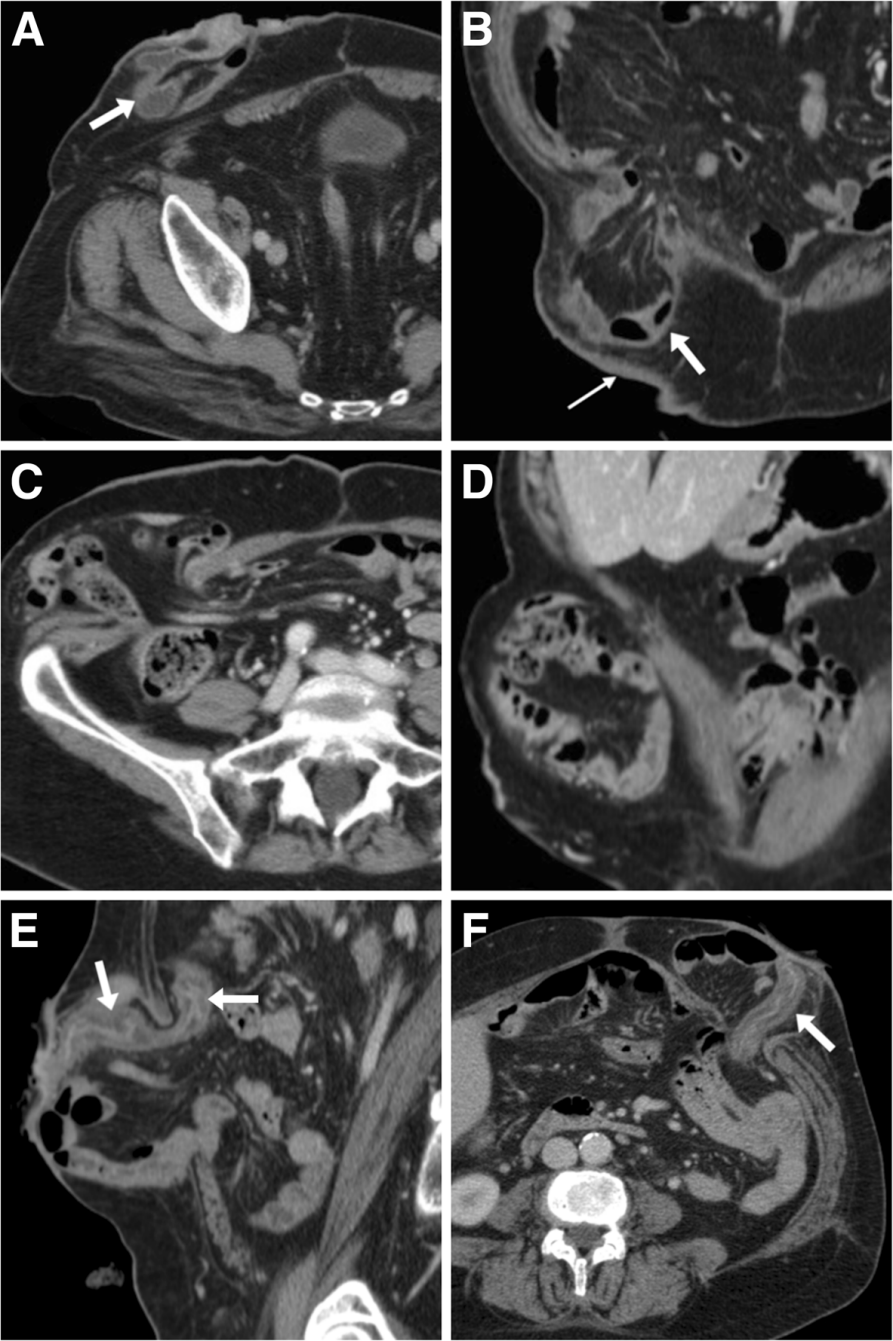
Three cases of uncomplicated PH. a, b CT type Ib PH measuring approximately 6 cm in size containing a redundant ileostomy tract (arrows). c, d Larger PH containing a large portion of the right colon. e, f Type III PH following recent Hartmann’s surgery containing the oedematous colostomy tract (arrows) and some small bowel loops
